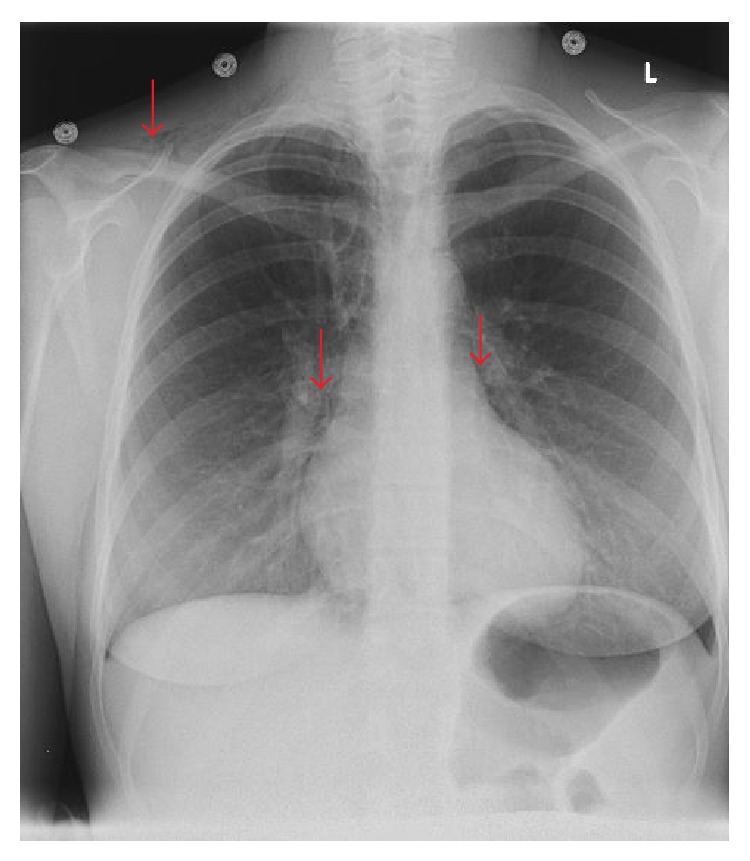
Just prior to discharge, a chest X-ray shows marked improvement in subcutaneous emphysema (red arrows).
